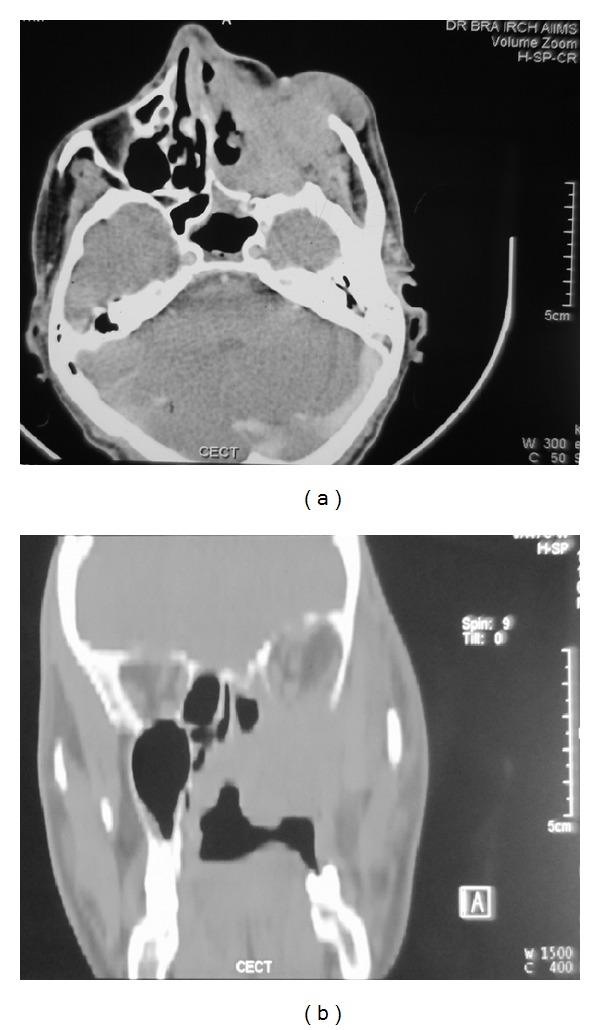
Axial (a) and coronal (b) images of contrast enhanced CT of the paranasal sinuses show an ill-defined poorly enhancing lesion at the operated (maxillectomy) site extending into the left orbit.
